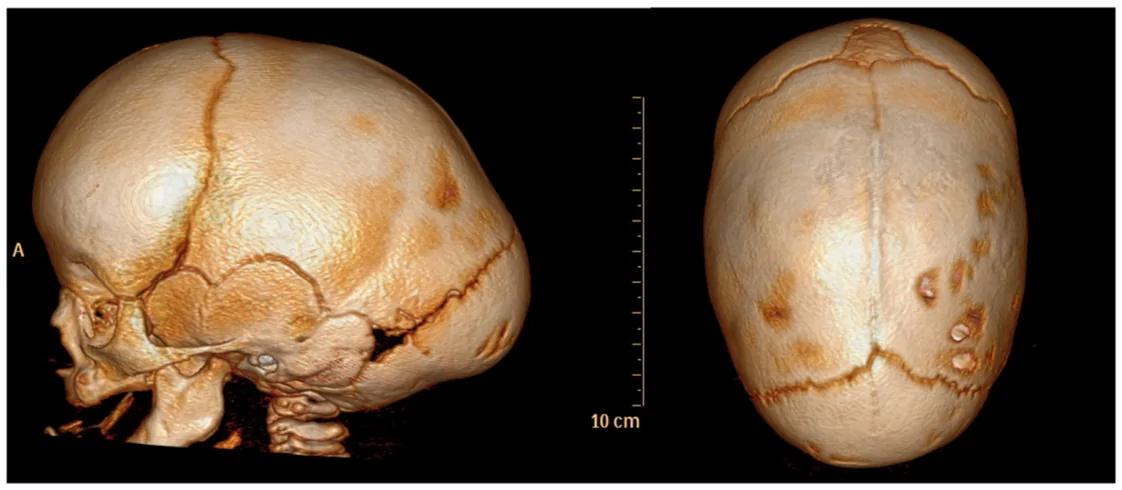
Craniosynostosis on computed tomography. Computed tomography (CT) with 3D reconstruction showing scaphocephaly due to closure of the sagittal suture. (left) Lateral view. (right) Superior (vertex) view showing the ridge along the fused sagittal suture.
